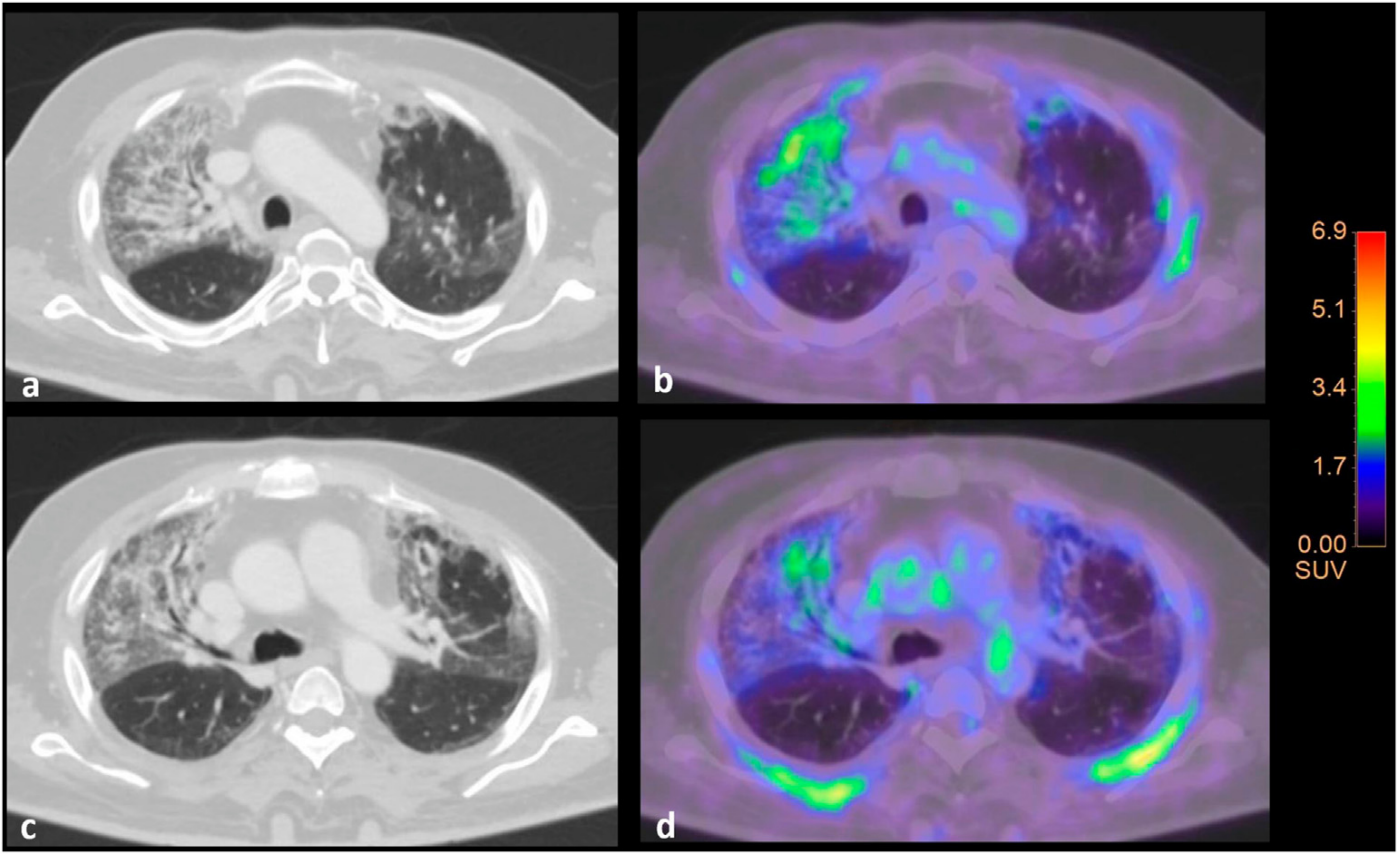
2-[^18^F]FDG PET/CT in patient of Figure 3 demonstrating diffused bilateral lung abnormalities (CO-RADS 5) (a and c) with increased inhomogeneous 2-[^18^F]FDG activity (SUV max 5.1) (b and d) as outcome imaging findings of interstitial pneumoniae by prior COVID-19 infection.
